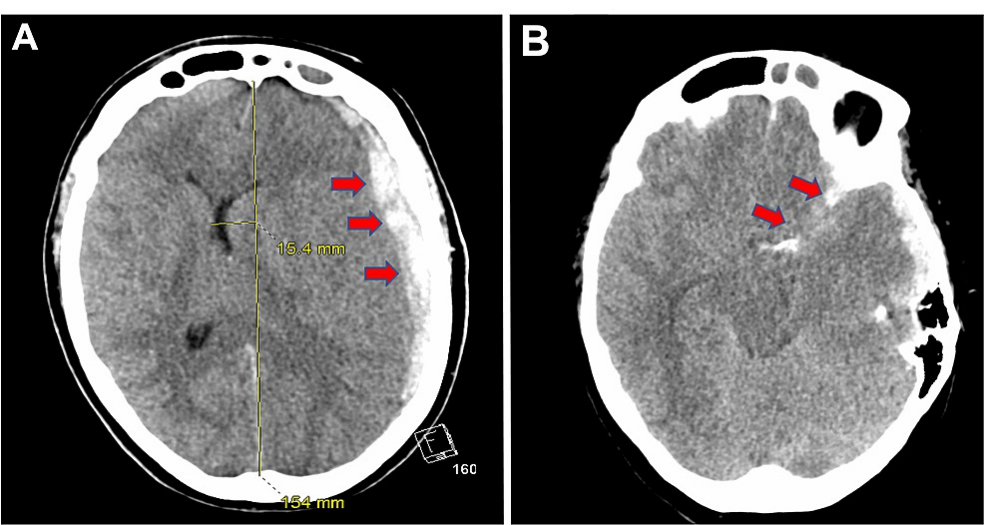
CT Scan of the Head A. Left hemispheric subdural hematoma resulting in mass effect, left-to-right midline shift, and brain herniation (red arrows). B. Scant subarachnoid hemorrhage in the Sylvian fissure (red arrows).
